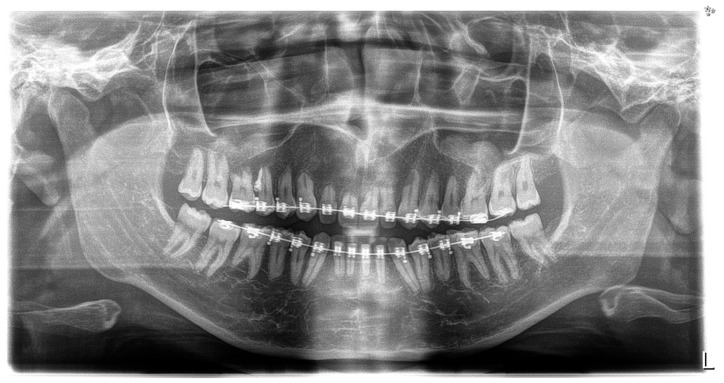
Panoramic radiograph at the end of the treatment. Severe root resorption was observed.
